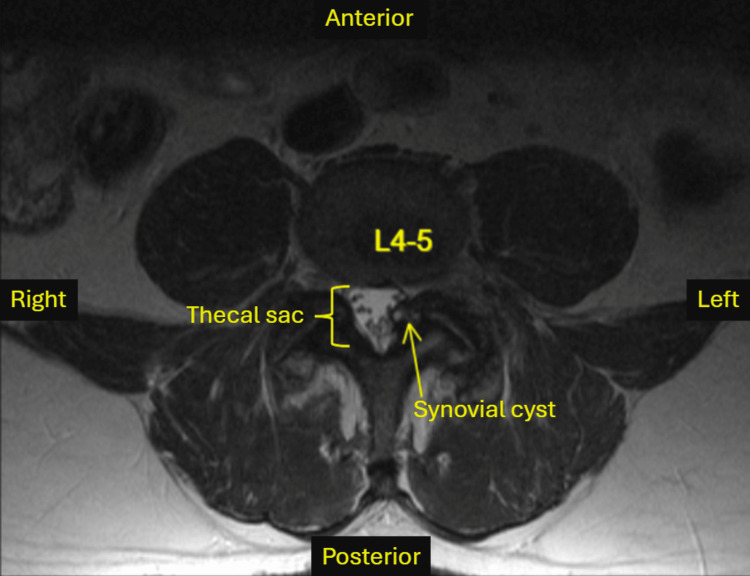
Preoperative lumbar spine MRI Demonstrates the L4-5 left synovial cyst indenting the thecal sac, without causing neural impingement.
